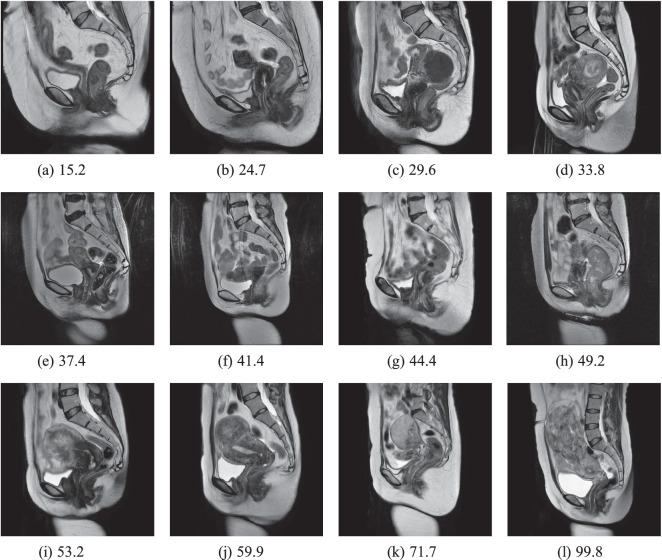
Case examples for different SNR. The SNR keeps getting higher from left to right and from top to bottom(the larger the SNR value, the higher the quality of the MRI).
